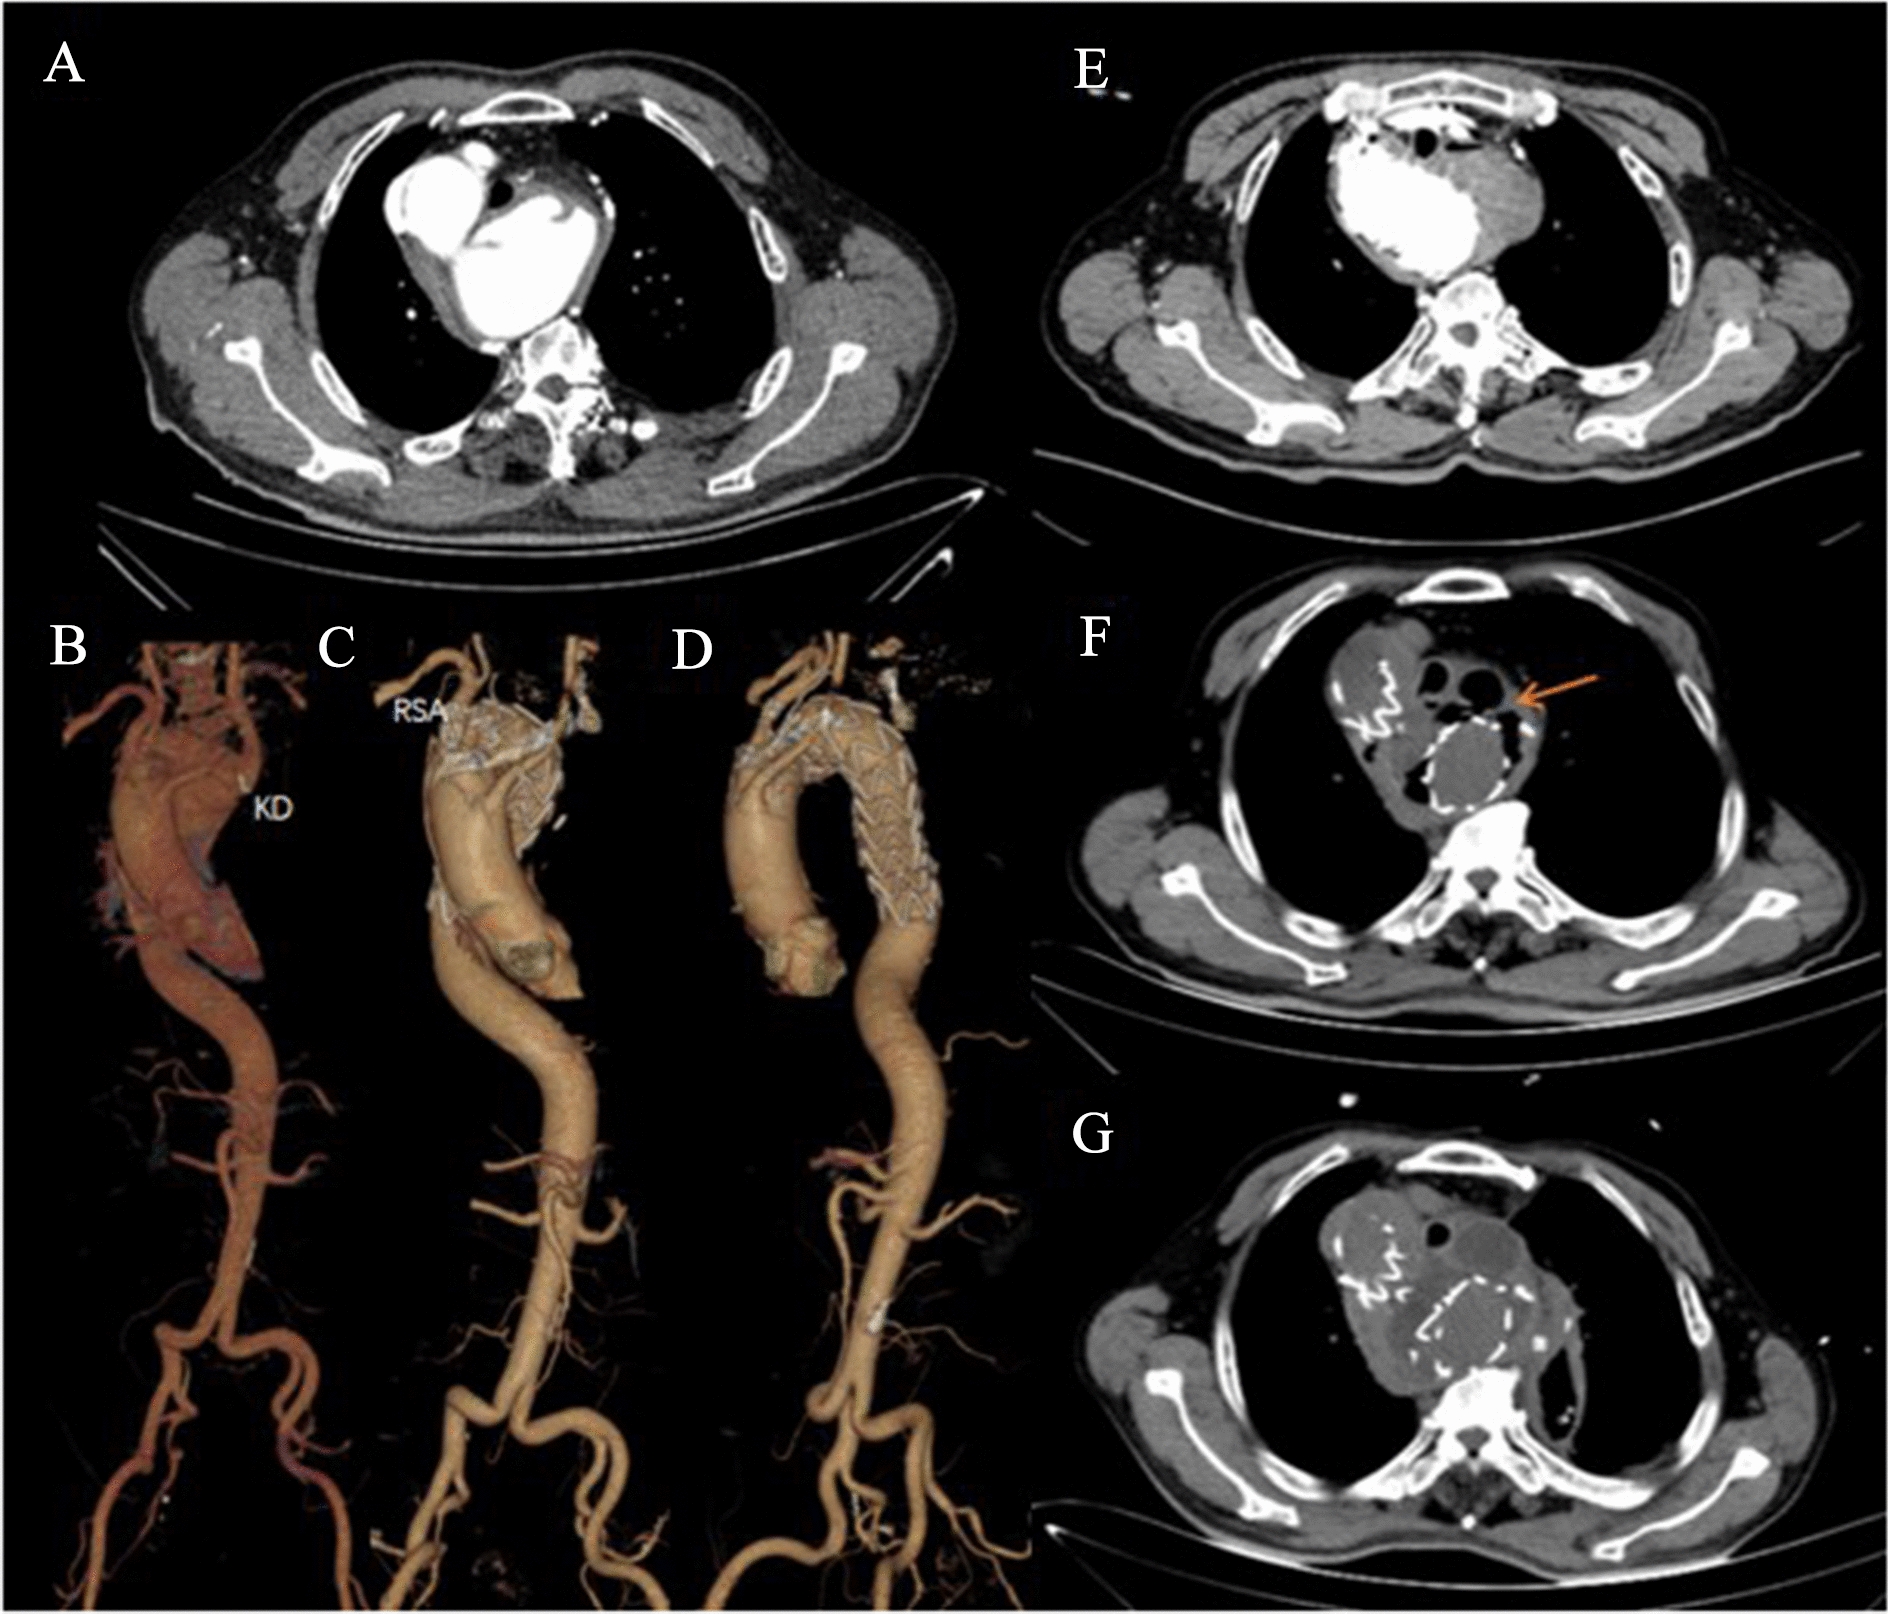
Postoperative complications in patient 3. CT scan and three-dimensional reconstruction image of postoperative computed tomographic angiogram (A–D) and Patient 3 developed postoperative esophageal fistula complications: 1 week after castor stent implantation (E). Esophageal and mediastinal fistula (orange arrow) occurred 1 month after operation (F). CT scan after gastroesophagostomy (G)
